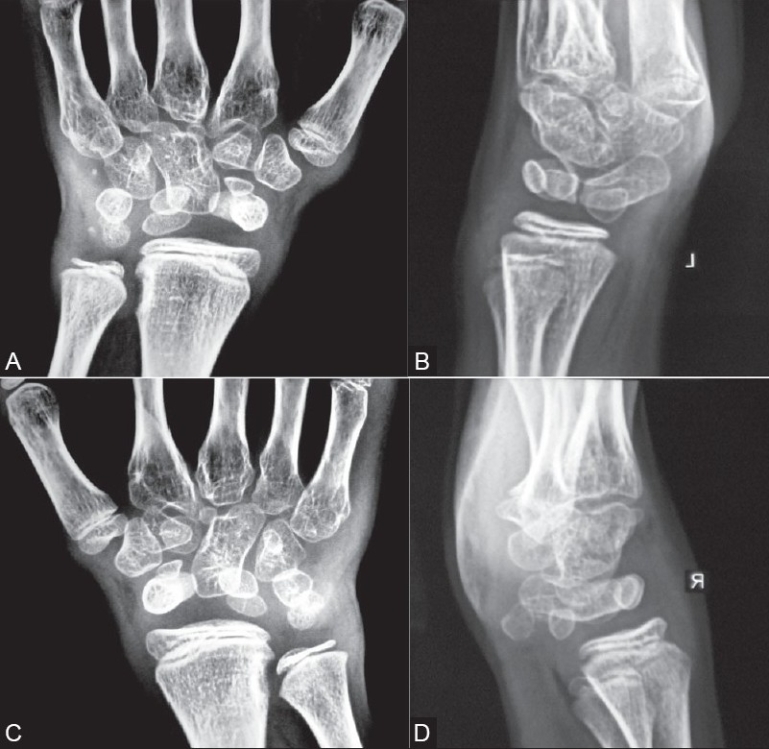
Frontal and lateral radiographs of the hands show extra carpal bones, 15 on the left (A, B) and 13 on the right (C, D), along with negative ulnar variance and impingement of the lower end of the ulna upon the medial cortex of the lower end of the radius. Note the swelling of the wrists due to the abnormal lie and position of the carpal bones
